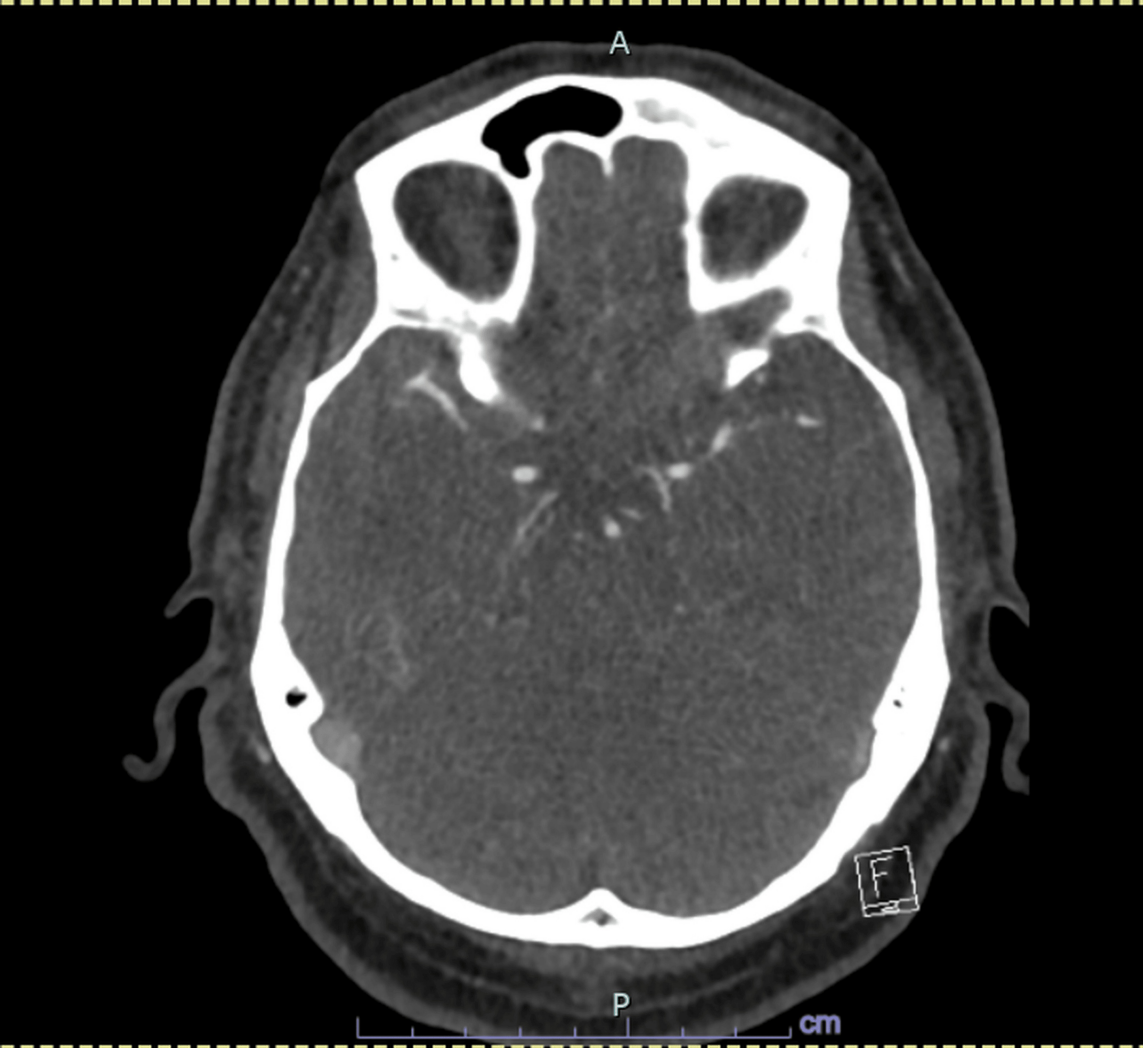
Axial CT angiography of the head at the level of the Circle of Willis demonstrating patent intracranial arteries with no evidence of large vessel occlusion
